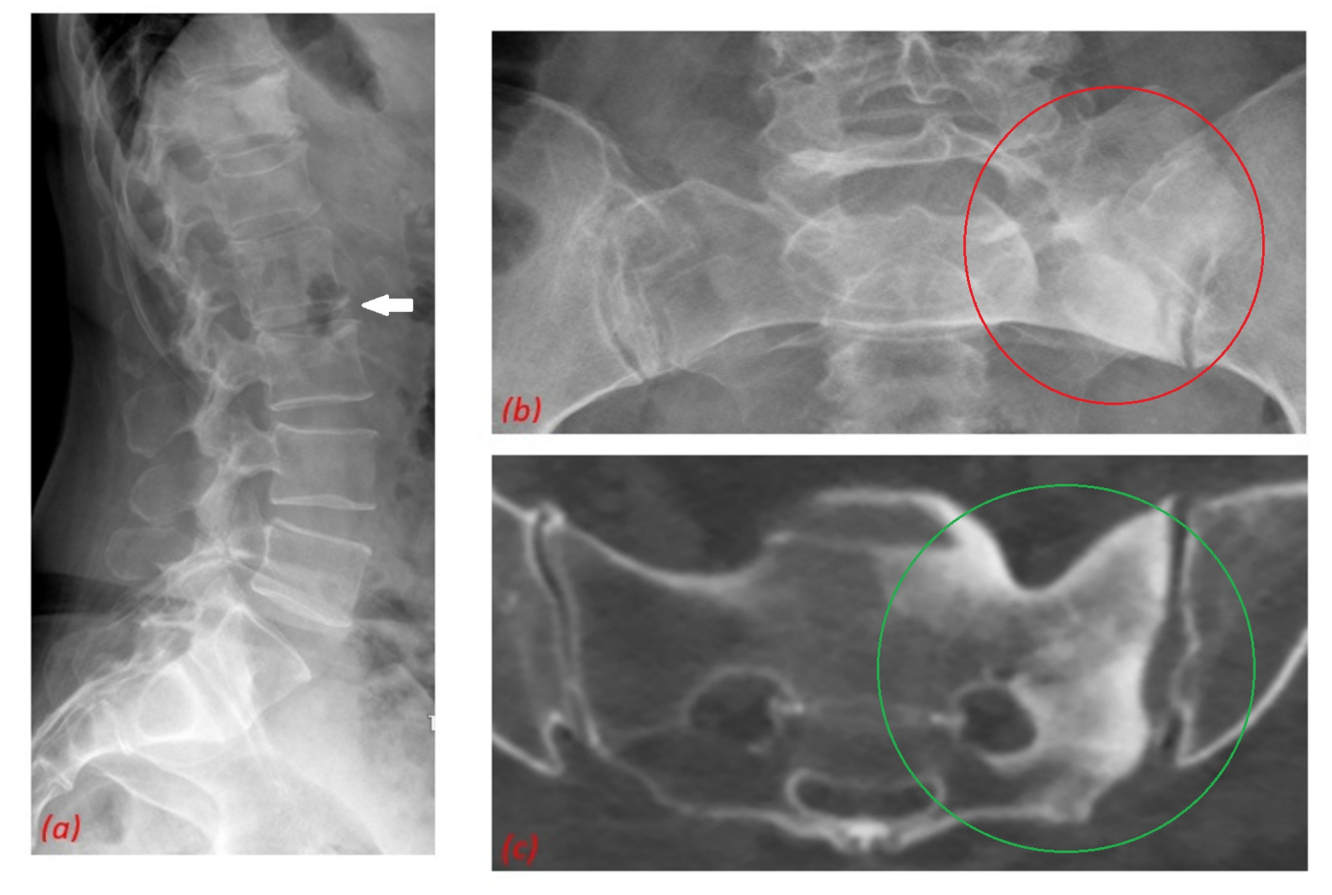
(a) X-ray of the spine shows compression fracture of L3 vertebrae (white arrow). Both X-ray (orange circle) (b) and CT scan (green circle) of the (c) pelvis show multiple areas of sclerosis on the left side of sacrum.
